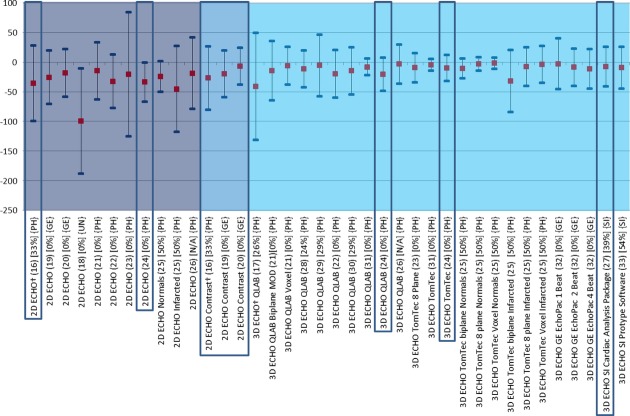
Comparison of echocardiographic techniques with cardiac magnetic resonance imaging for measurement of end-systolic volume (mL). Red square box indicates bias compared with magnetic resonance imaging. Blue line at each end of the plots indicates the lower and upper limits of agreement calculated by Bland–Altman. MRI = magnetic resonance imaging; 2D ECHO = two-dimensional echocardiography; 3D ECHO = three-dimensional echocardiography; NSR = normal sinus rhythm; MOD = method of disks; QLAB = Philips online and offline LV volume calculation tool; TomTec = offline left ventricular volume calculation tool.
